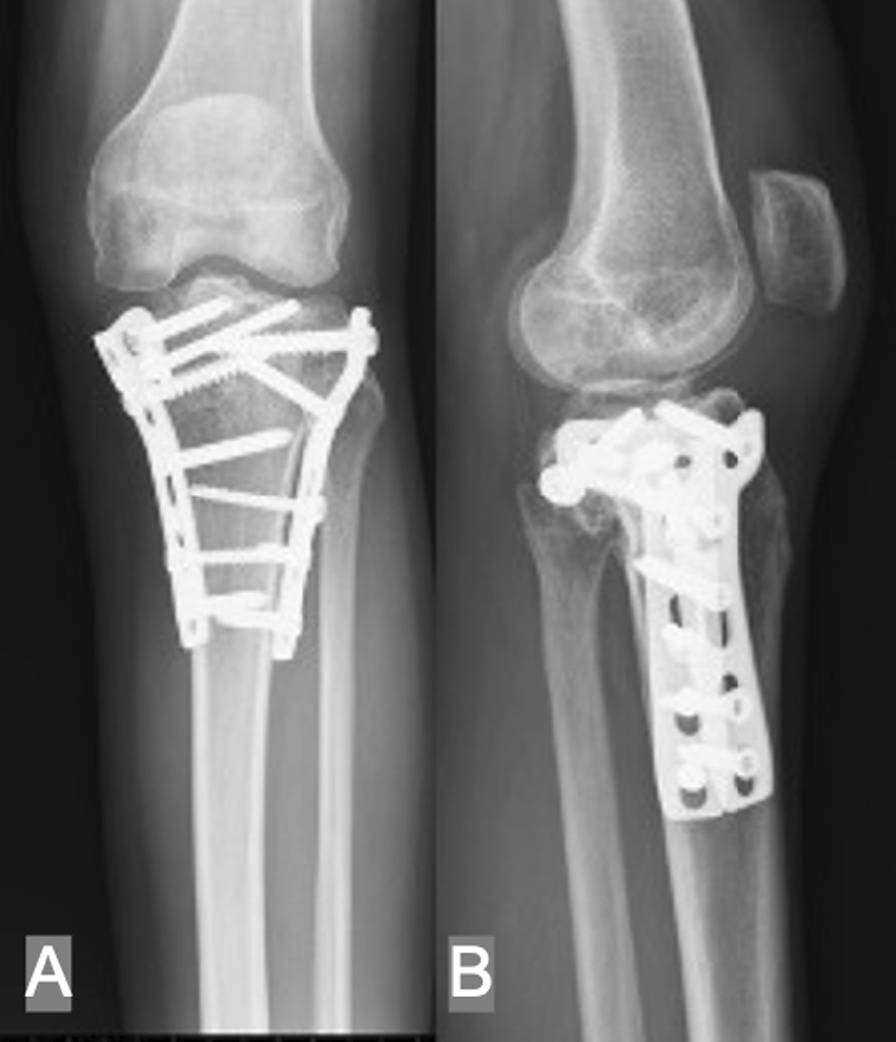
Radiography of the same patient mentioned above 3 years postoperatively. A, B Both planes of radiographic alignment were well maintained
